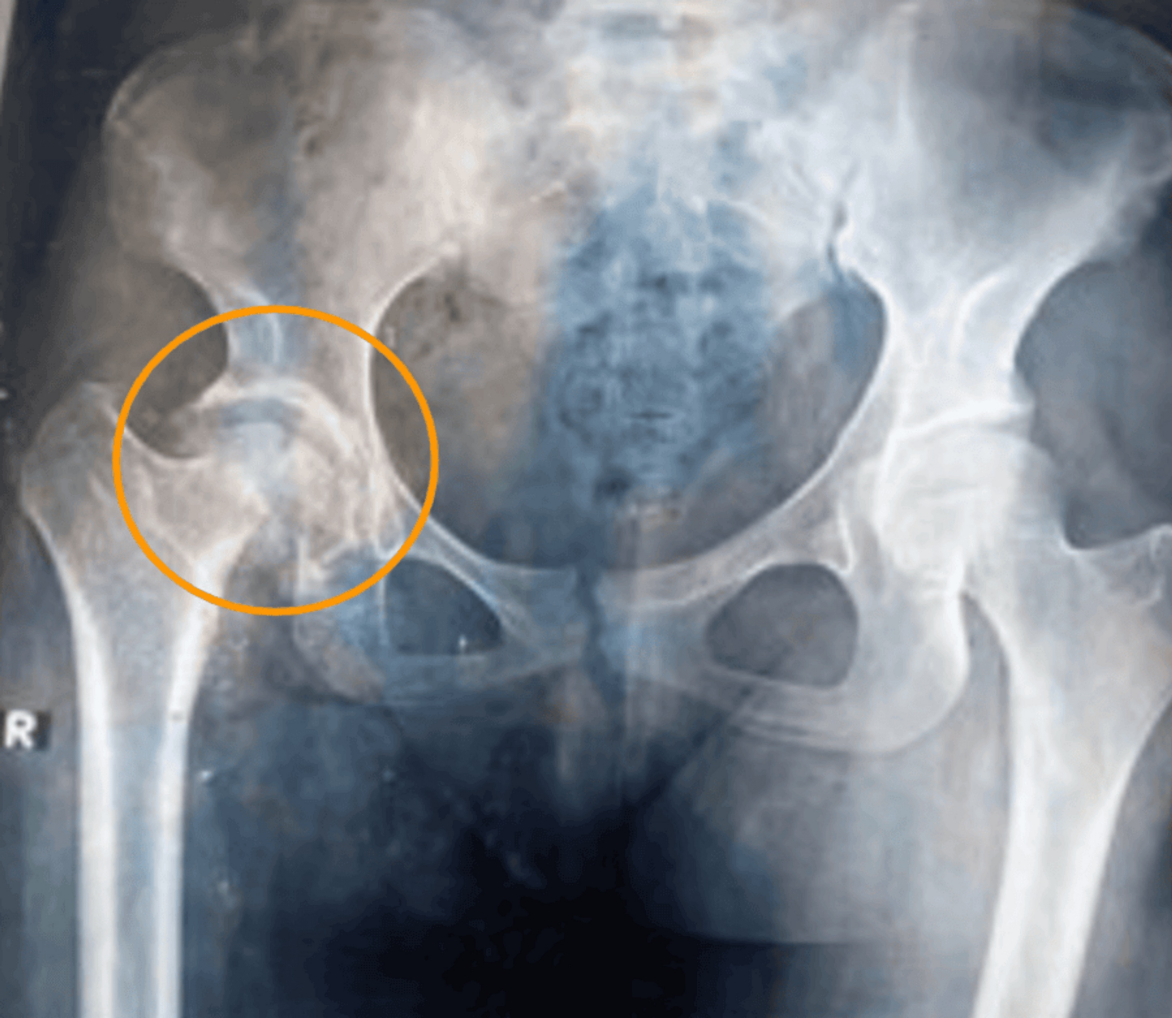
Frontal radiograph of pelvis including both proximal femurs. Avascular necrosis of right femoral head with reduced head volume and widened acetabulum. Early development of synovial osteochondromatosis was also evident showing few calcified loose bodies. The yellow circle encompasses the AVN of the right femoral head with reduced head volume and widened acetabulum. Early development of synovial osteochondromatosis was also evident, showing a few calcified loose bodies. AVN: Avascular necrosis
